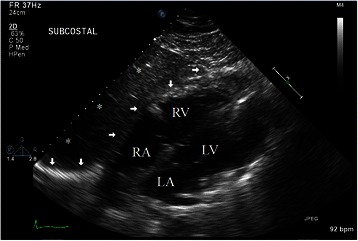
Subcostal view Transthoracic Echocardiogram. This subcostal view demonstrates the extra-pericardial accumulation of blood encompassing the majority of the anterior heart. Arrows: Borders of hematoma, Asterisks: Hematoma, RA: Right atrium, RV: Right ventricle, LA: Left atrium, LV: Left ventricle
